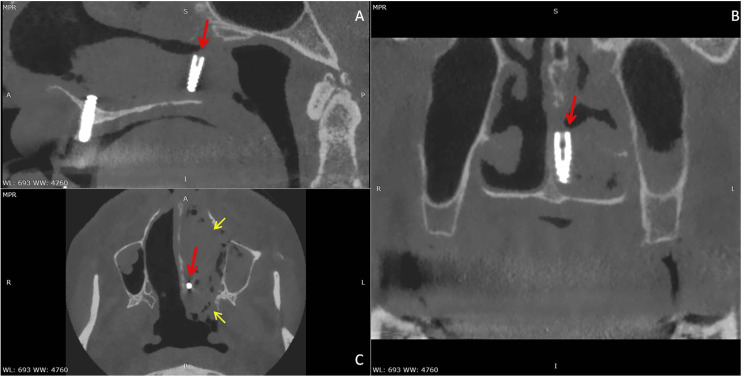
CT scan (A: sagittal, B: coronal, and C: axial views). A cylindrical, hyperdense foreign body measuring approximately 11 × 4 mm situated beneath the tail of the left inferior nasal concha, extending posteriorly toward the choana (red arrow). Evidence of hemorrhagic collection within the left nasal cavity and the ipsilateral maxillary sinus (yellow arrow). CT: computed tomography
